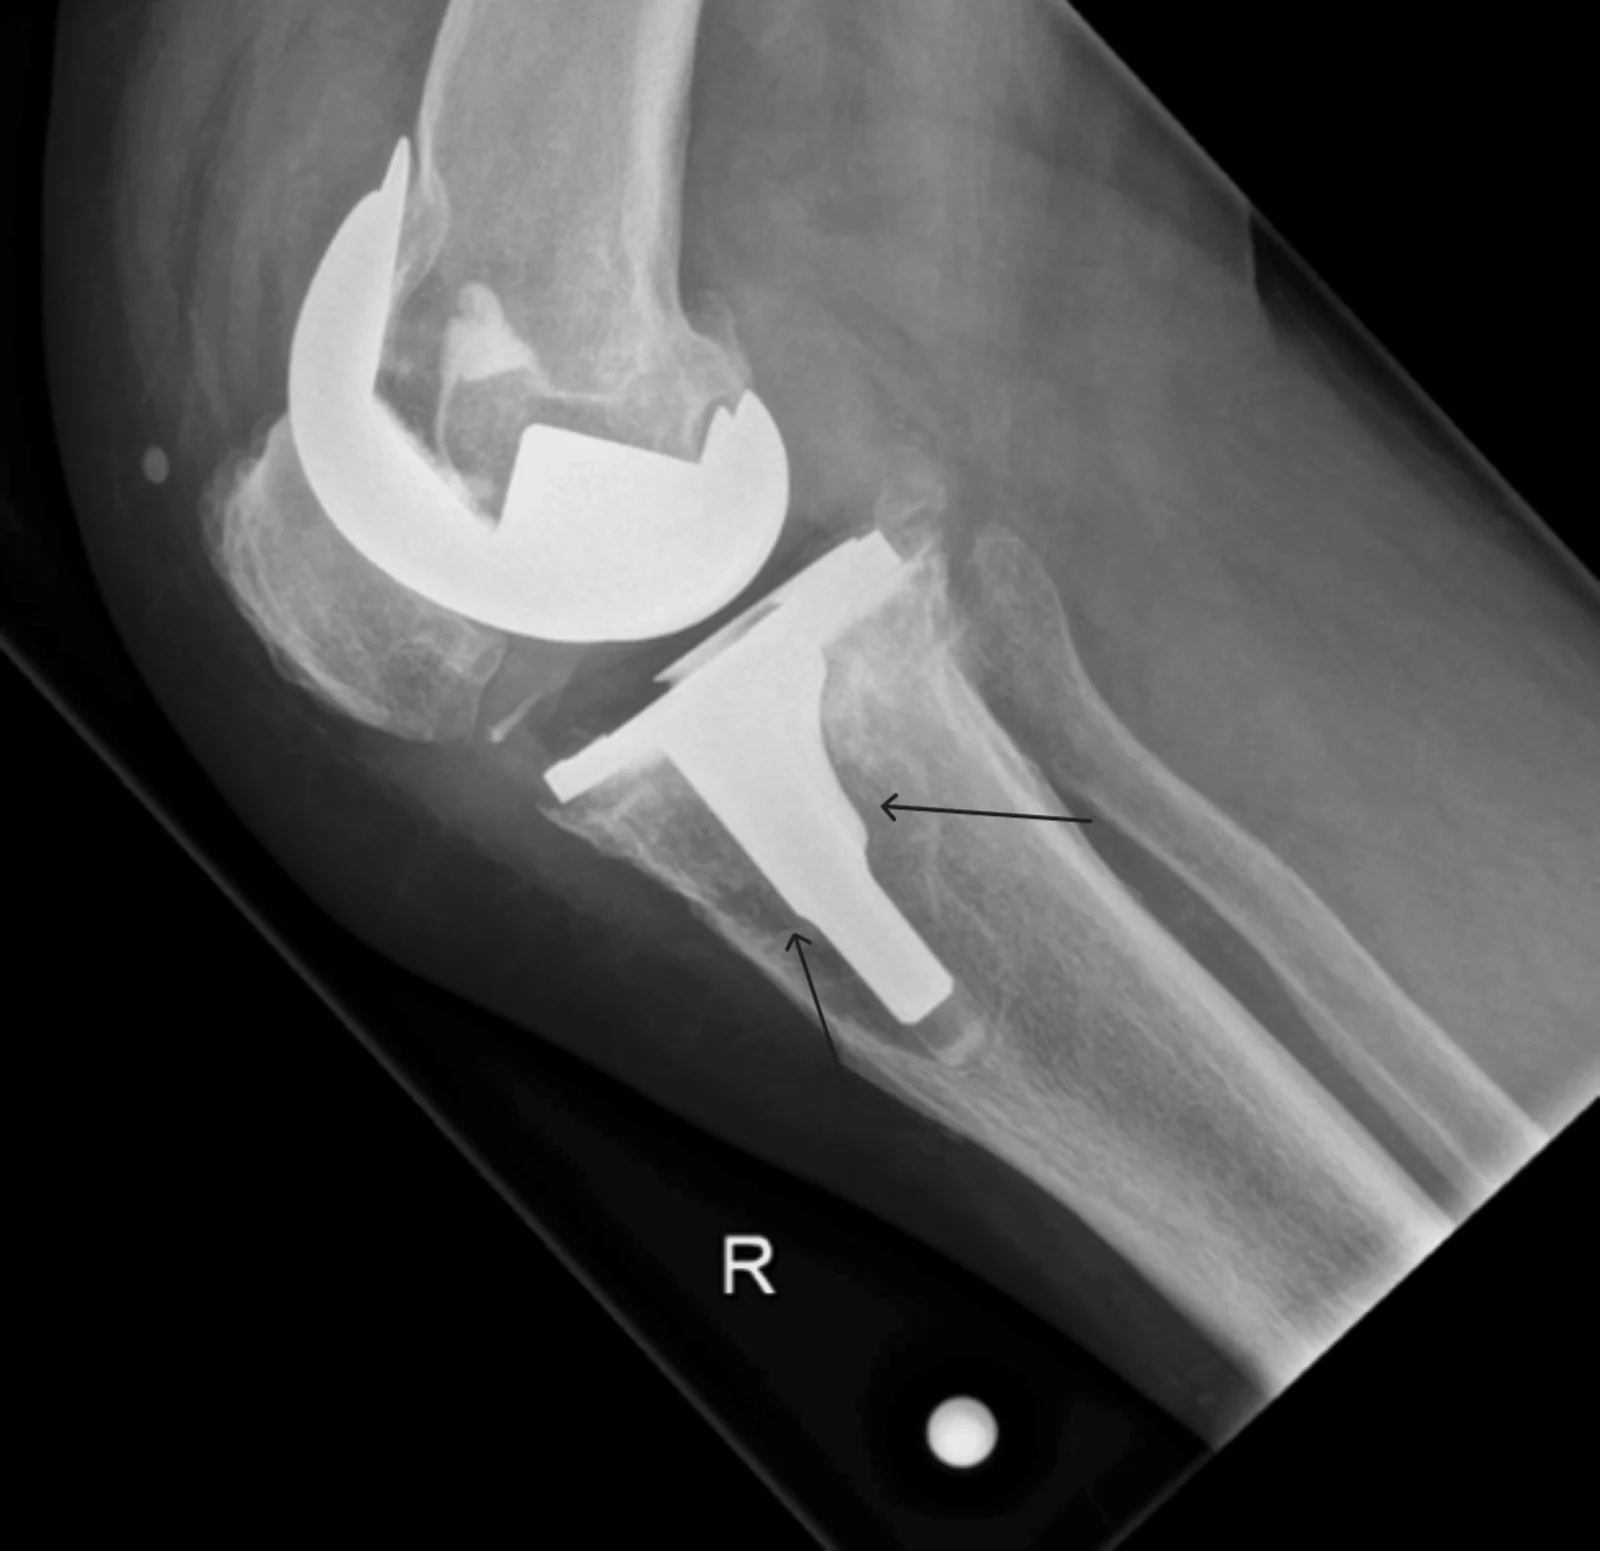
Lateral radiograph of the right knee showing minimal signs of osteolysis surrounding the TKA five years after primary TKA. TKA, total knee arthroplasty
